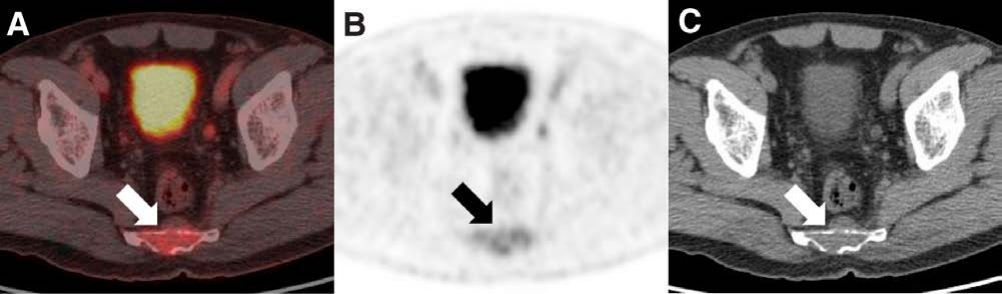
A 68-year-old man who had sacral chordoma. Axial (A) PET/CT, (B) PET, and (C) CT images show a 6.7 cm mass in the sacrum with a visual score of 2 and heterogenous uptake. The tumor-to-liver ratio (TLR), maximum standardized uptake value (SUVmax), and total lesion glycolysis (TLG) were 1.7, 5.1, and 48.3, respectively. The mass was proven as sacral chordoma after excision. CT = computed tomography, PET = positron emission tomography, TLR = tumor-to-liver ratio.
